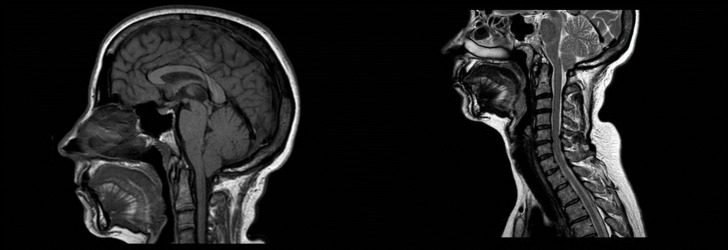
Brain MRI showing Chiari I malformation Brain MRI T1 (left) and T2 (right) weighted sagittal sequences show a Chiari I malformation. The tonsillar descent is about 8-8.5 mm. MRI: magnetic resonance imaging
